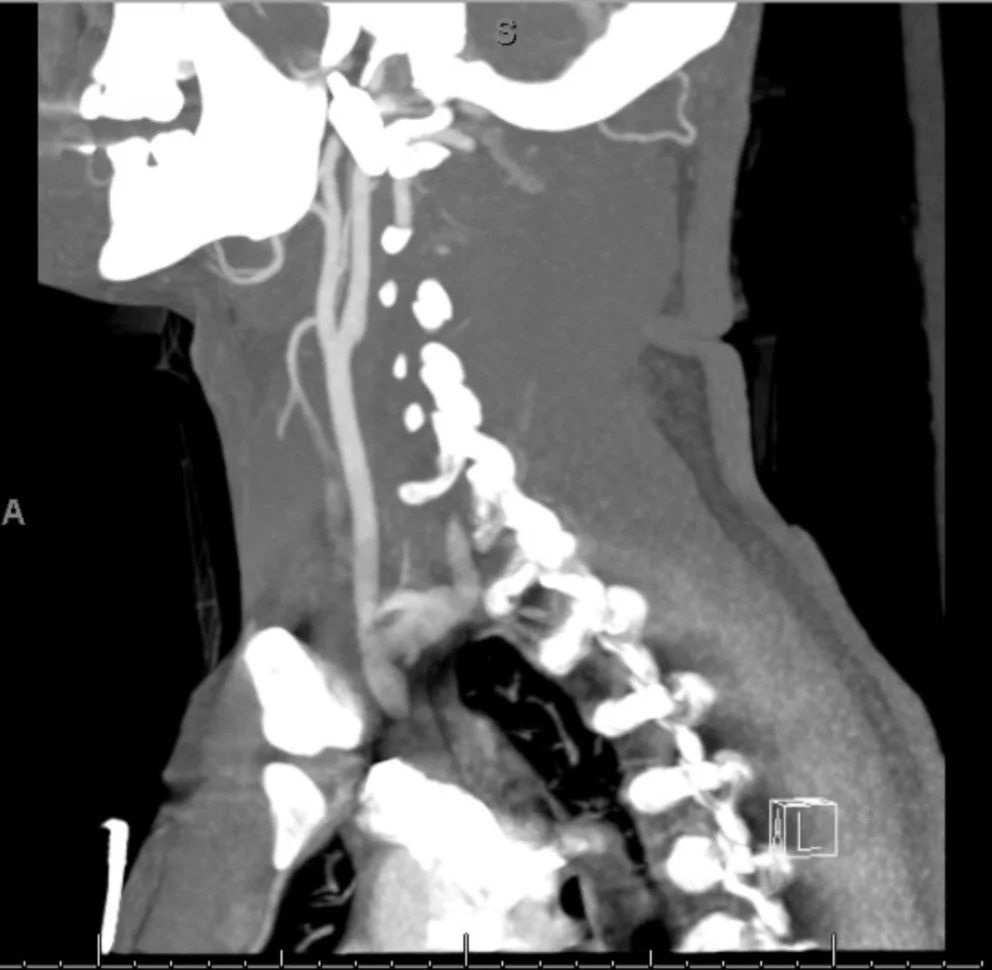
Sagittal CTA neck image showing foreign body in right parapharyngeal space.
